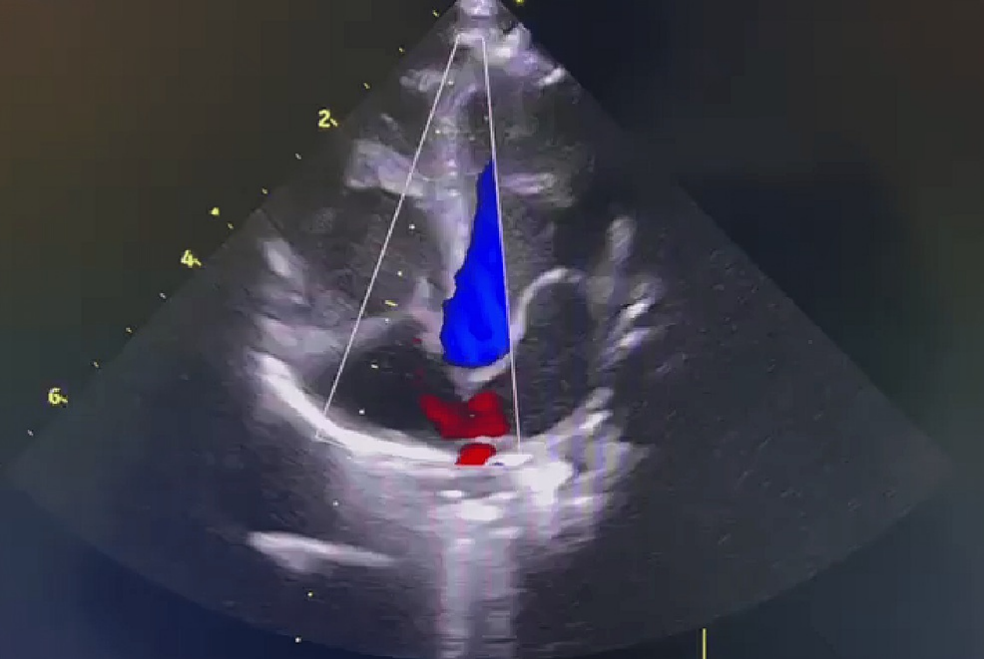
Echocardiogram of case number one Echocardiogram of case number one showing a small ostium secundum-type interatrial communication with an exclusive left-to-right shunt
